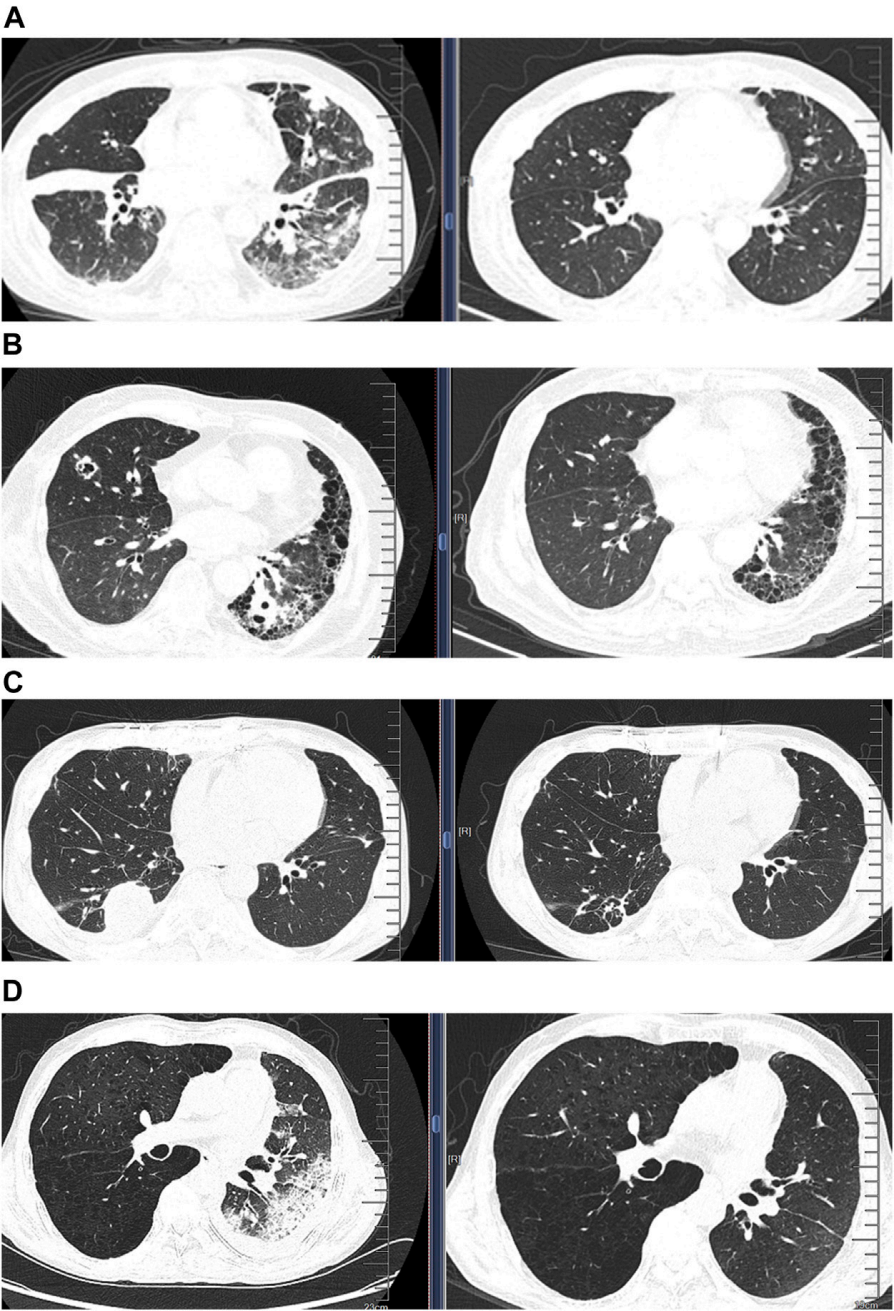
Comparison of chest computed tomographic (CT) images before and after treatment in four patients whose treatment regimens were switched thoroughly according to the mNGS findings. (A) CT images from a patient diagnosed as having *Pneumocystis jirovecii* pneumonia according to mNGS; CT images showing significant improvement of infiltration after treatment (right) compared with that before treatment (left); (B) CT images of disseminated nocardiosis before and after treatment; (C) CT images of NTM pulmonary disease before and after treatment; (D) CT images of acute rejection before and after treatment.
